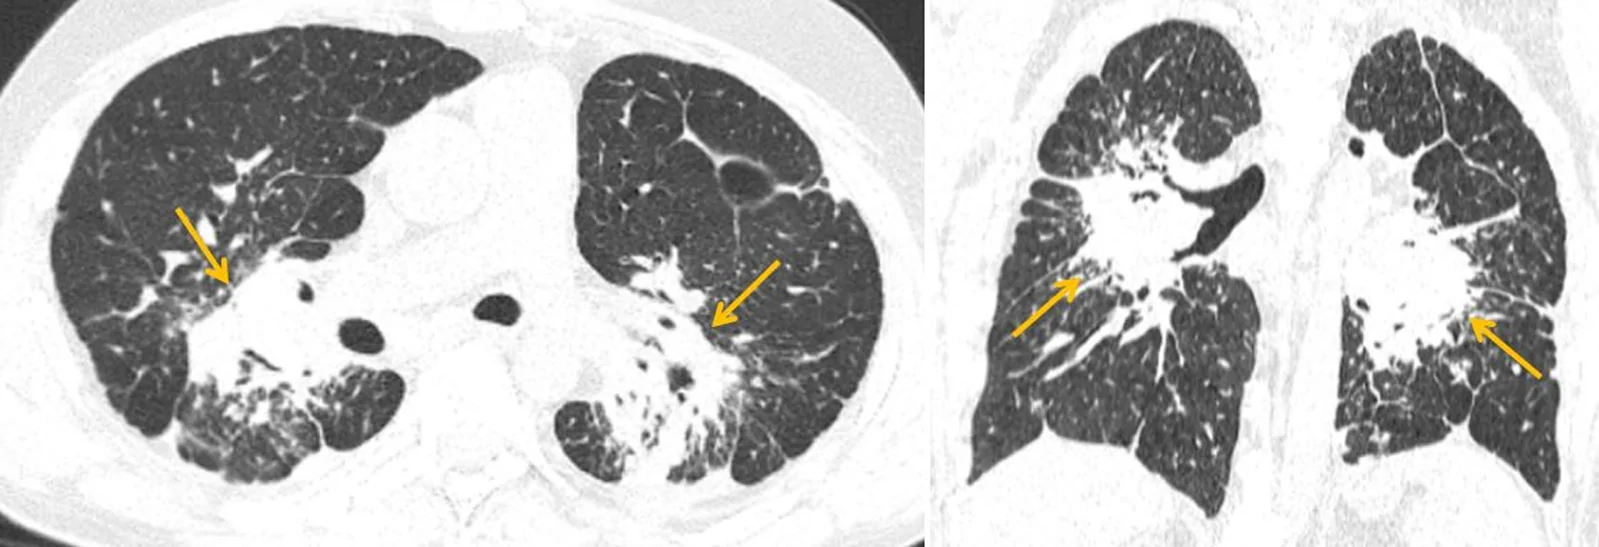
Axial and coronal HRCT images (lung window) demonstrating heterogeneous perihilar consolidation with airless conglomerates (yellow arrows), preserved bronchial patency, and linear pleural adhesions. HRCT: high-resolution computed tomography.
